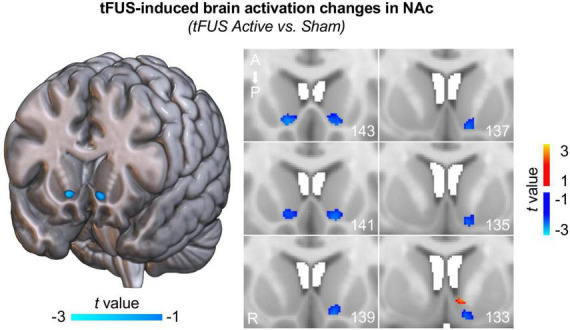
Transcranial focused ultrasound (tFUS) induces inhibited brain activation in NAc. Decreased brain activities were observed in the anterior part of the bilateral NAc and most regions of the left posterior NAc (two-sample *t*-test, *p* < 0.05, uncorrected) in the tFUS active group compared to the sham group, indicating that tFUS inhibited NAc activities. Note that, these deactivation maps were estimated based on group data comparison between active group and sham group within the NAc. Each figure on the right panel depicts different slices through the striatum. The numbers in the bottom right corners represent the slice number; ascending numbers go from anterior to posterior.
